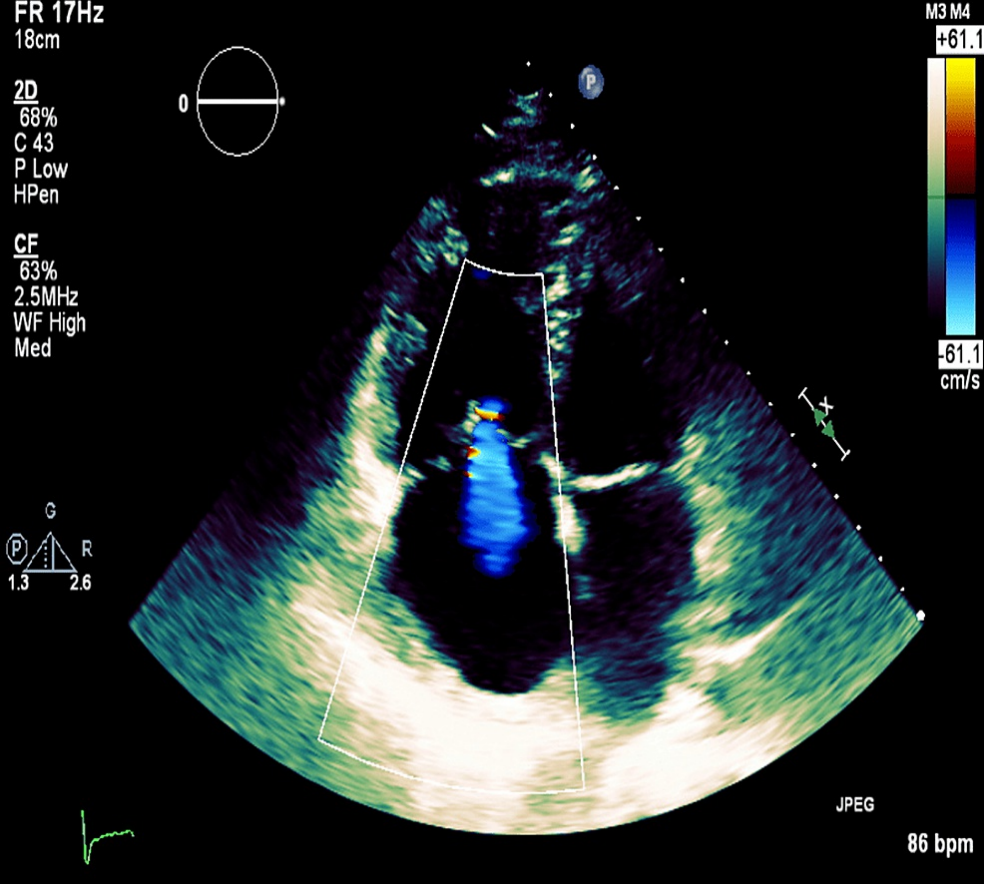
Transthoracic echocardiogram Transthoracic echocardiogram Four chamber view shows severe dilatation of right heart cavities.
